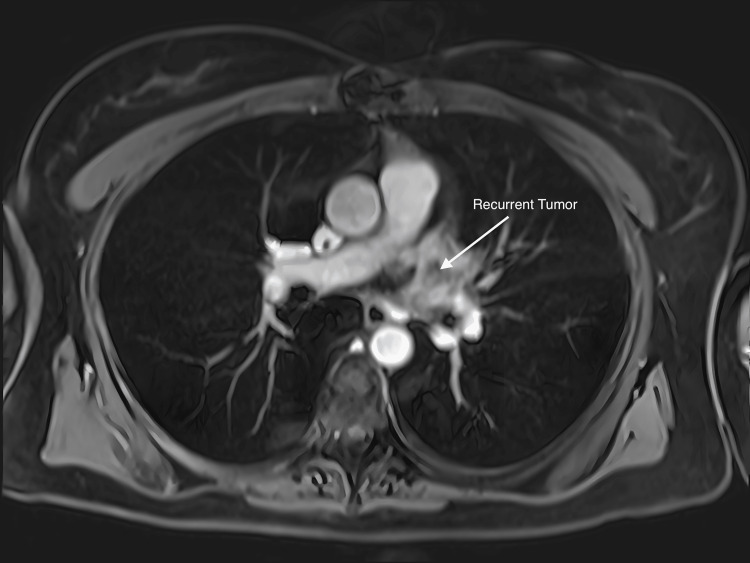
Axial MRI scan on 3/25/19 demonstrating a recurrent atrial mass.
